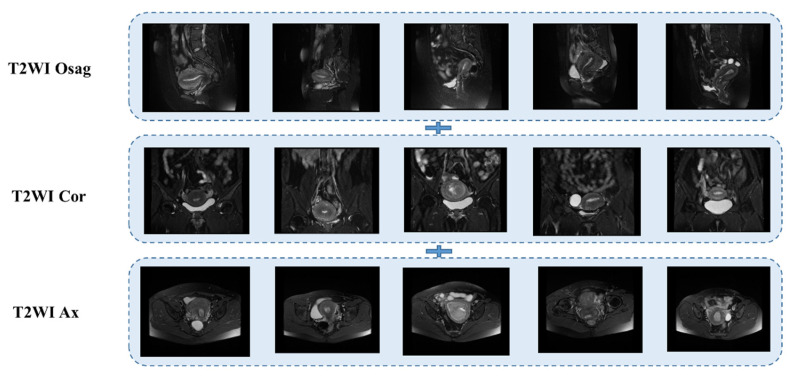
MRI T2WI sequence of endometrial cancer.
